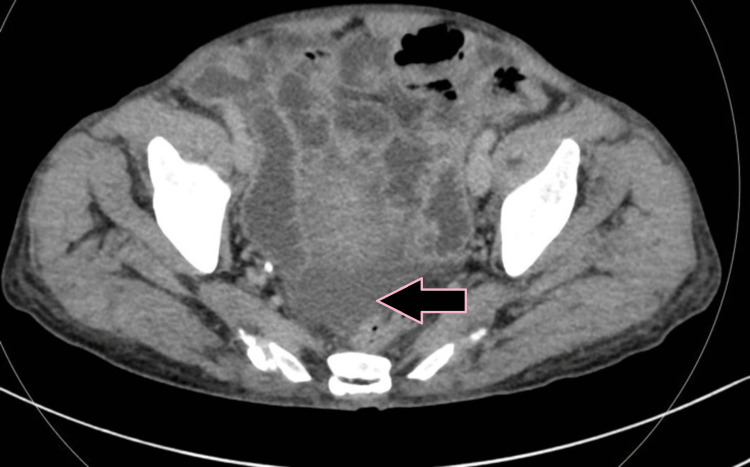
Axial MDCT with contrast showing ascites in the peritoneal cavity (case 2). MDCT: multidetector computed tomography
